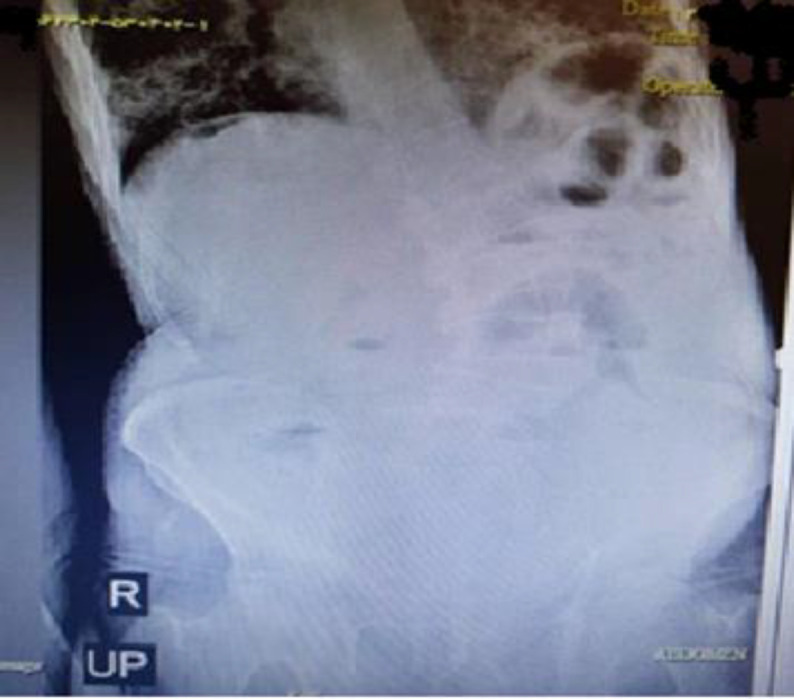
Abdominal X-ray, upright and supine
